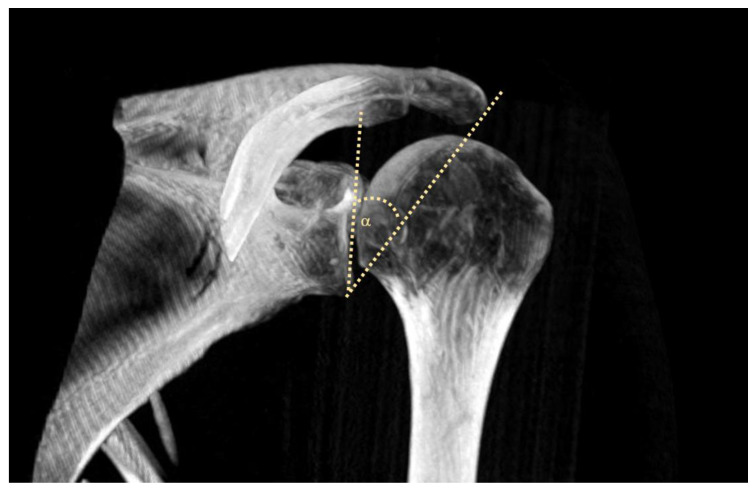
Three-dimensional reconstruction of a CT scan of the shoulder joint with drawn CSA.
